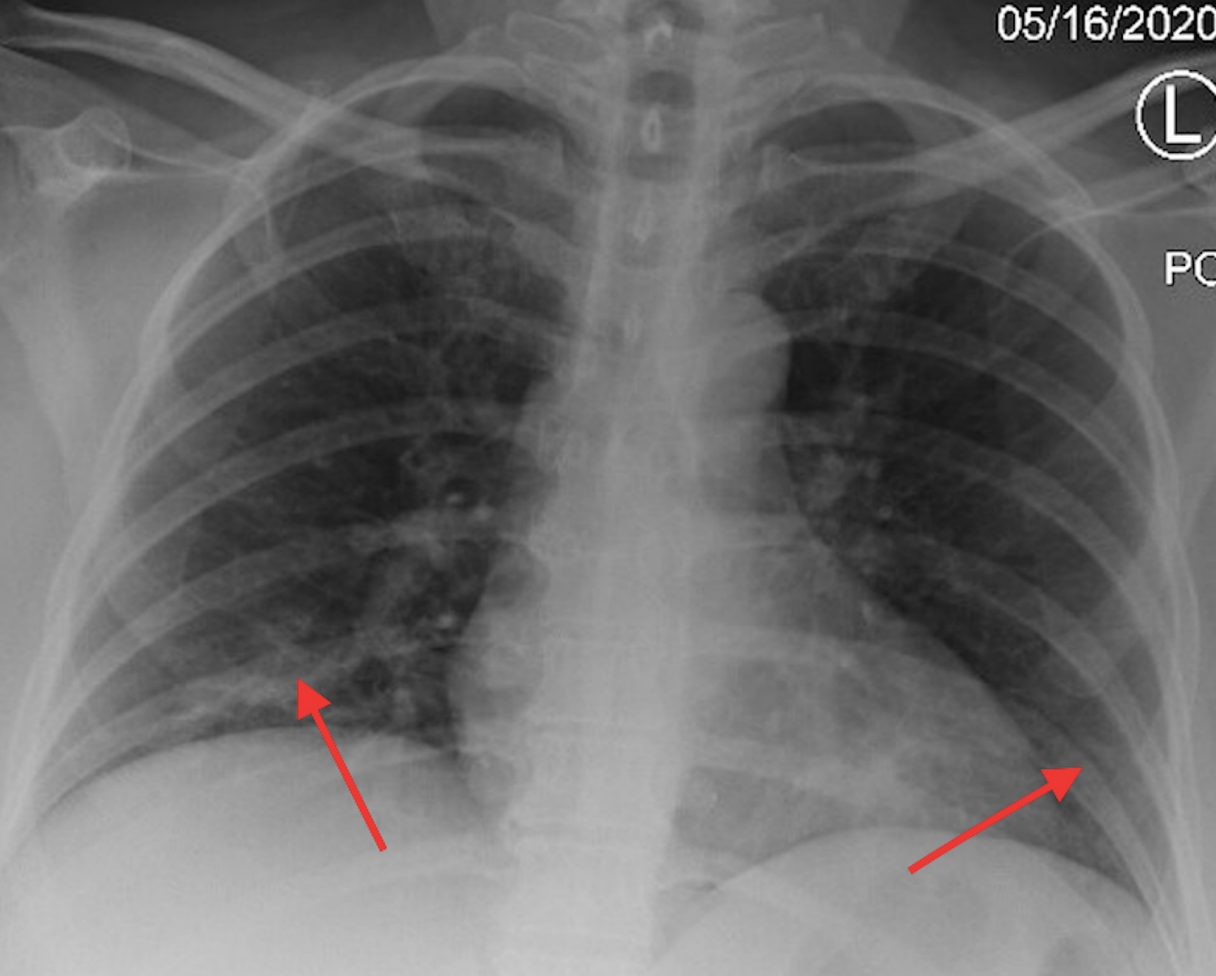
Chest x-ray on the day of admission showed mild bilateral patchy airspace opacities, nonspecific but compatible with a reported history of coronavirus disease 2019 (COVID-19) infection (red arrows)
